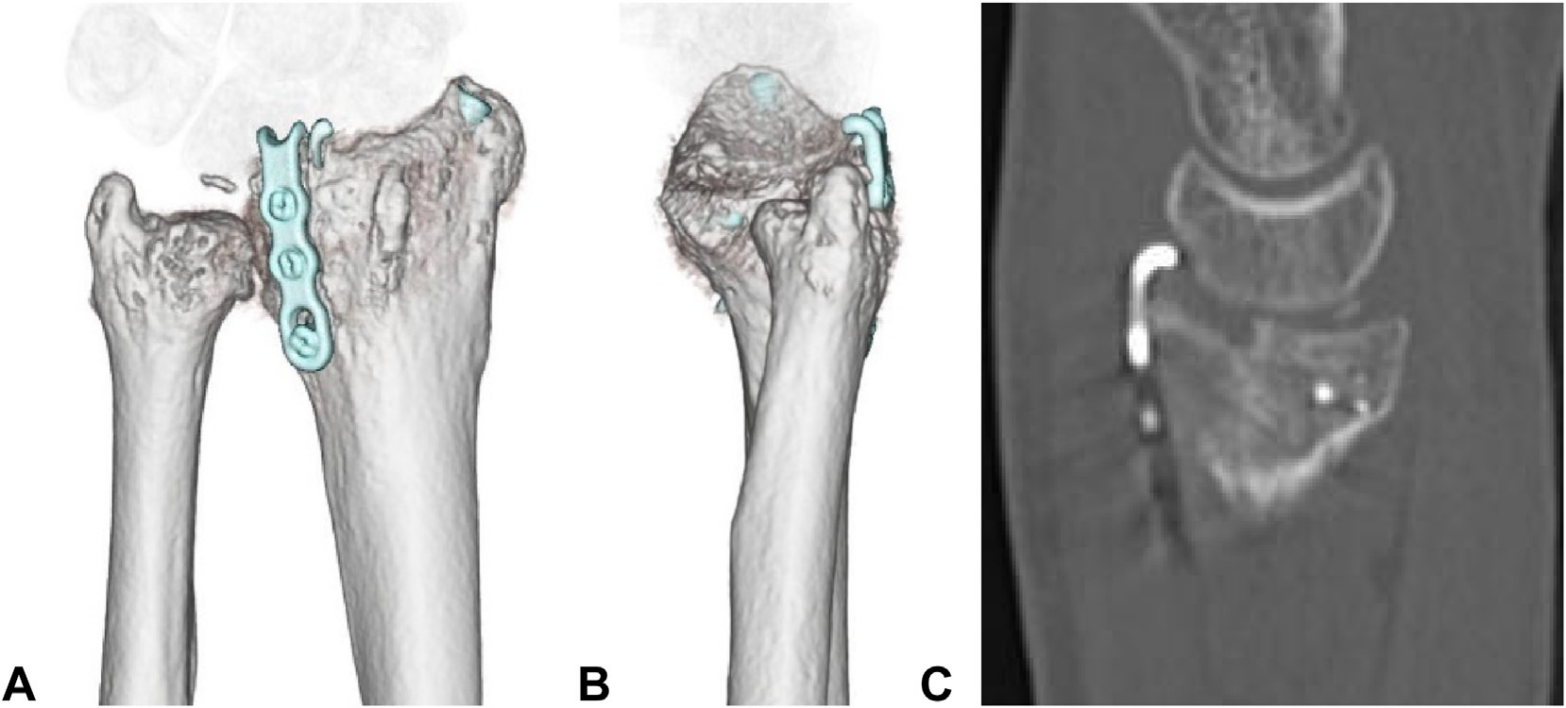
Images showing graft union and good articular surface congruency. A Post-transplant dorsal view. B Post-transplant ulnar view. C Computed tomography sagittal reformation.
